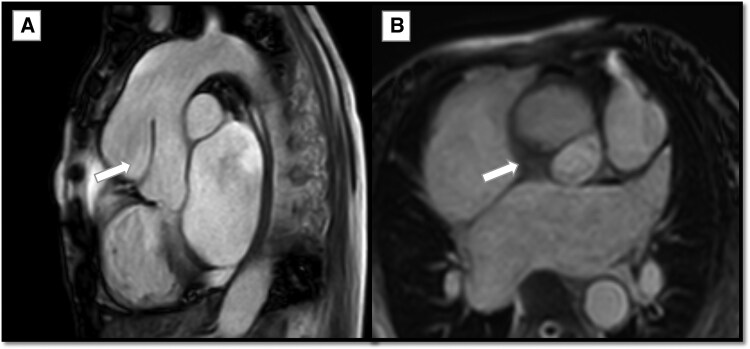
Cardiovascular magnetic resonance imaging SSFP. (*A*) Sagittal cut SSFP image showing retrograde Stanford Type A aortic dissection (thick arrow). (*B*) Axial cut SSFP image showing circumferential, crescentic soft tissue mass encasing the ascending aorta just above the level of sinotubular junction with true and false lumens.
